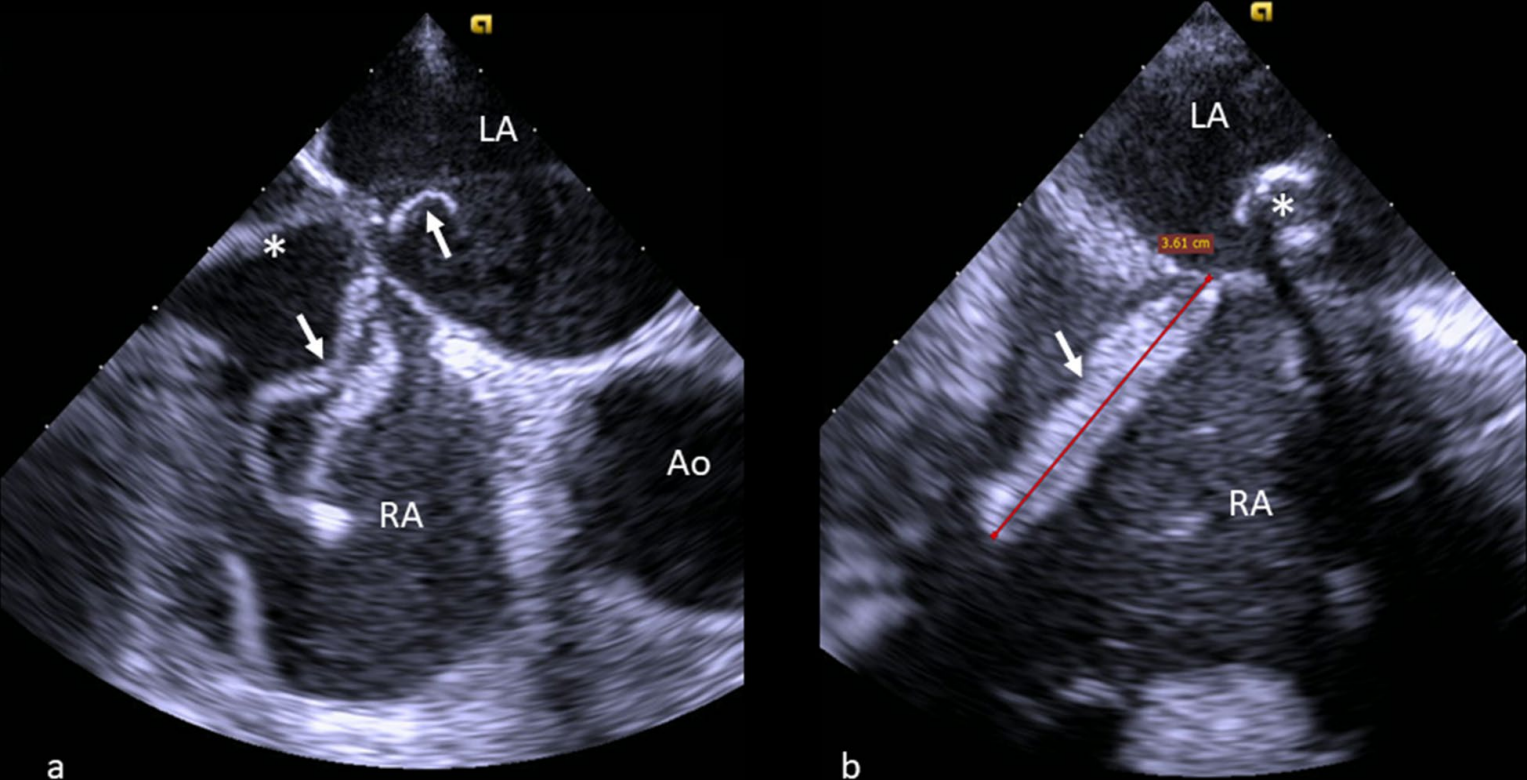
A Transesophageal echocardiography, ME AV SAX view. B Transesophageal echocardiography, ME bicaval view. LA: left atrium, RA: right atrium, Ao: aorta, * steerable guidance catheter, arrow: thrombus
